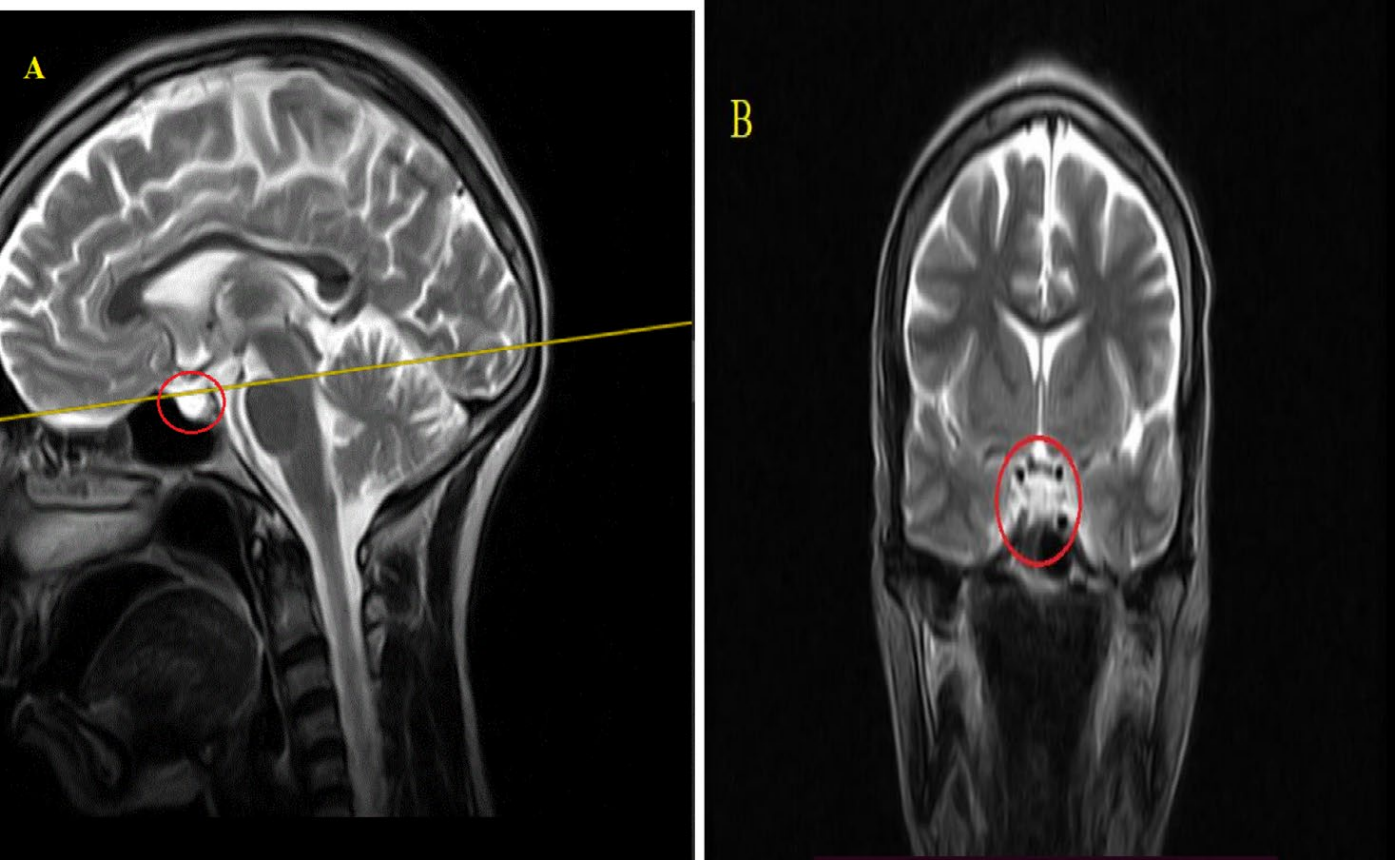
Additional T2‐weighted MRI views of the sellar region. (A) Sagittal view repeats anatomical context and confirms CSF‐filled sella space (red circle). (B) Coronal view again reveals minimal pituitary tissue with CSF predominance, consistent with significant pituitary atrophy posthemorrhage (red circle). CSF, cerebrospinal fluid.
